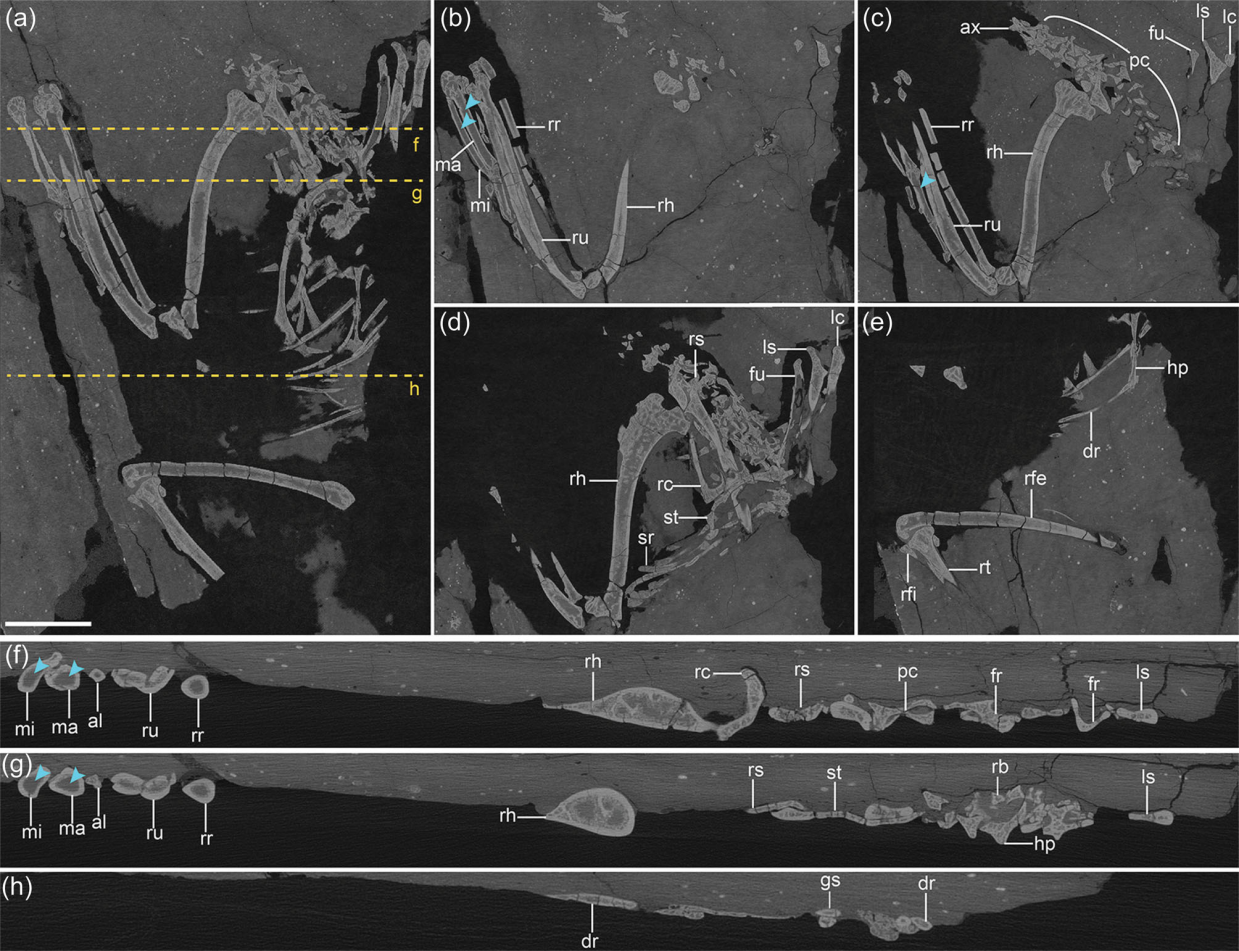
X-ray computed tomography imaging of *Mirusavis*, IVPP V18692. (a)–(e) Dorsal/ventral view of the full skeleton (a) and in different horizons ((b)–(e)), showing the distribution of medullary bone; (f)–(h) virtual cross sections (denoted by orange dashed lines in (a)) of individual elements showing the medullary bone in all elements but the metacarpals and digits (blue arrowheads). al, alular digit; ax, axis; dr, dorsal rib; fr, furcular ramus; fu, furcula; gs, gastralia; hp, hypocleidium of furcula; lc, left coracoid; ls, left scapula; ma, major metacarpal; mi, minor metacarpal; pc, post-axis cervical vertebrae; rc, right coracoid; rfe, right femur; rfi, right fibula; rh, right humerus; rr, right radius; rs, right scapula; rt, right tibiotarsus; ru, right ulna; sr, sternal rib; st, sternum. Scale bar equals 10 mm in (a); (b)–(h) are not scaled.
